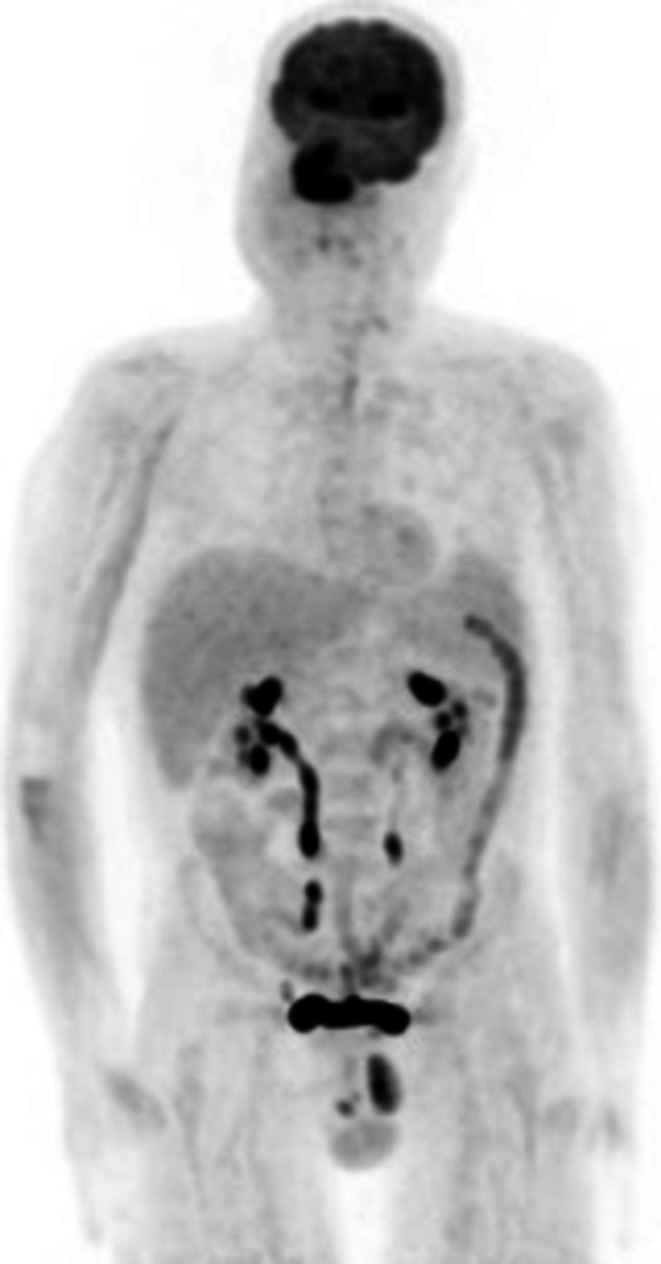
ENKTL of the nasal region. Whole‐body FDG‐PET study.
